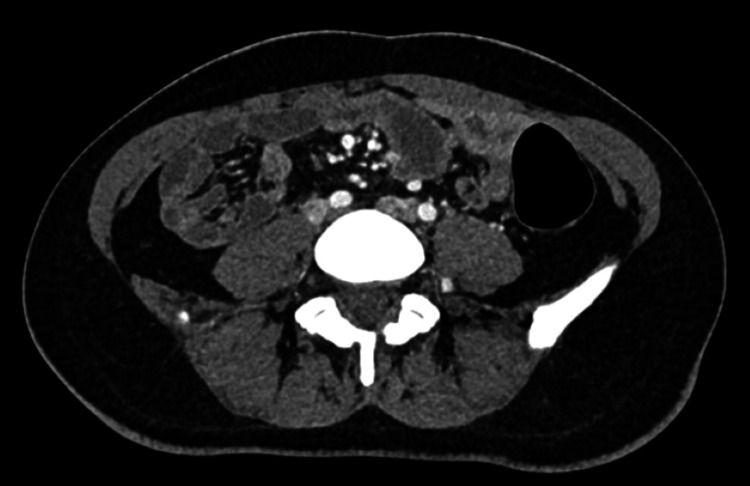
Axial CT of the abdomen. An axial contrast CT of the abdomen showing several mildly enlarged lymph nodes in the para-aortic region, adjacent to the abdominal aorta. The nodes are discrete and homogeneous in attenuation. There is no evidence of calcification or surrounding inflammatory change. These findings are suggestive of non-specific reactive lymphadenopathy.
